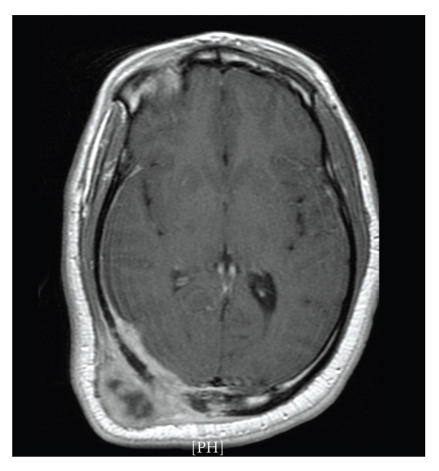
CT scan of the head shows a large vascular soft tissue mass involving the meninges and invasion of right parietal bone.
